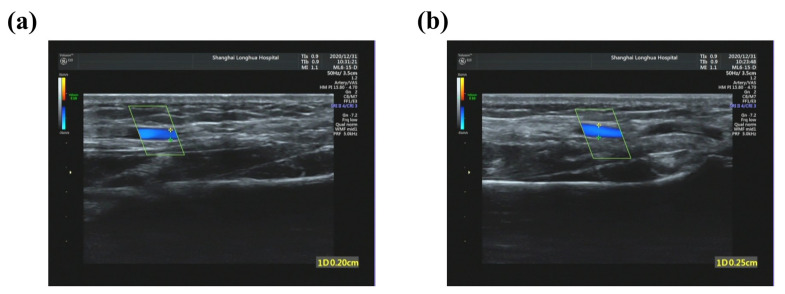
Diameter of Volunteer 1’s radial artery in diastole and systole was measured using an ultrasound instrument. (a) Diastolic diameter is 0.20 cm and (b) systolic diameter is 0.25 cm.
